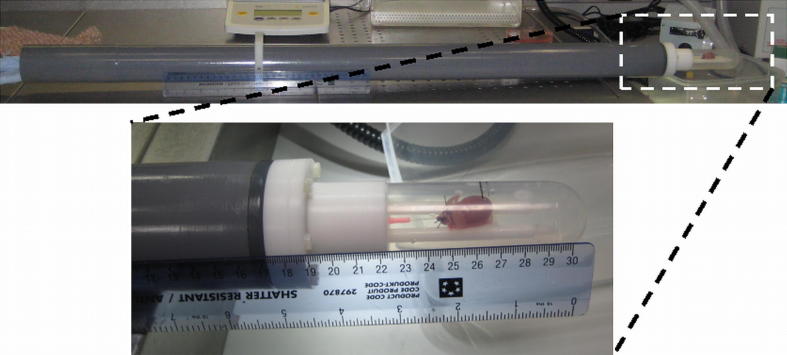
Photographs of the custom-made Langendorff perfusion rig. *Top*: view of a heart inside the imaging chamber, mounted to the rigid plastic support tube prior to insertion into the MRI scanner. The perfusate is carried through a water-jacketed line from the reservoir (not shown), through the support tube, and to the Langendorff-mounted heart in the chamber. Effluent solution leaves the system, via gravity, through the support tube. *Bottom*: magnified view of the heart chamber. Hearts were attached to an aortic cannula inside the chamber and placed on a flexible cradle made from Parafilm suspended between two support rods.
